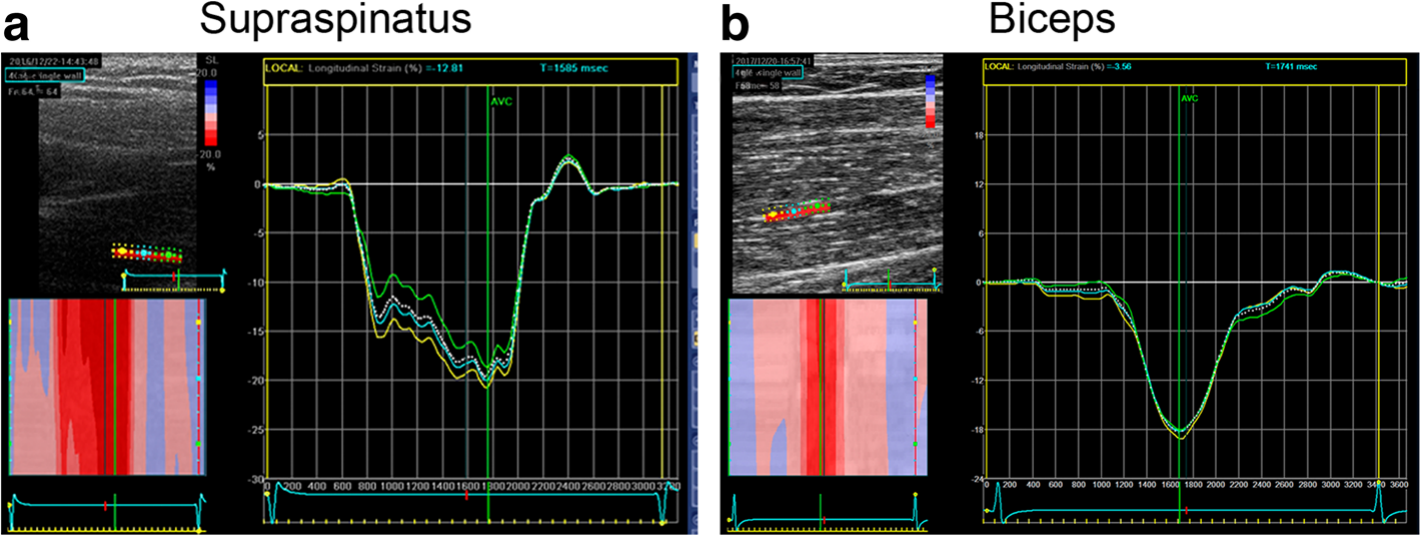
Supraspinatus and biceps muscle dynamics. a, b, Graphic depiction of speckle-tracking ultrasonographic analysis of supraspinatus (a) and biceps (b) showing representative measurements of longitudinal strain at 80 and 40% max load, respectively
